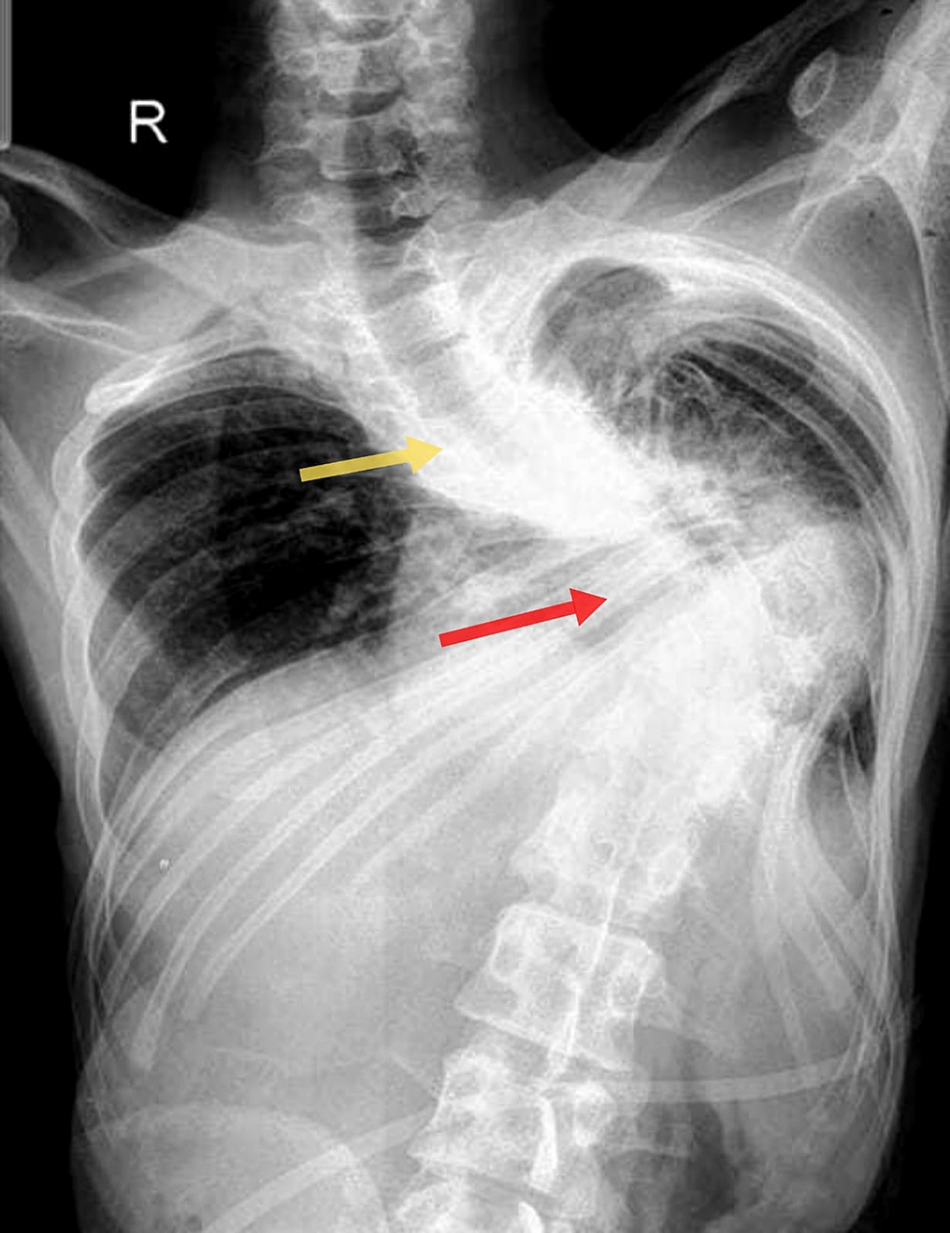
Chest X-ray shows tracheal deviation toward the left side of the chest (yellow arrow) and thoracic scoliosis (red arrow).
